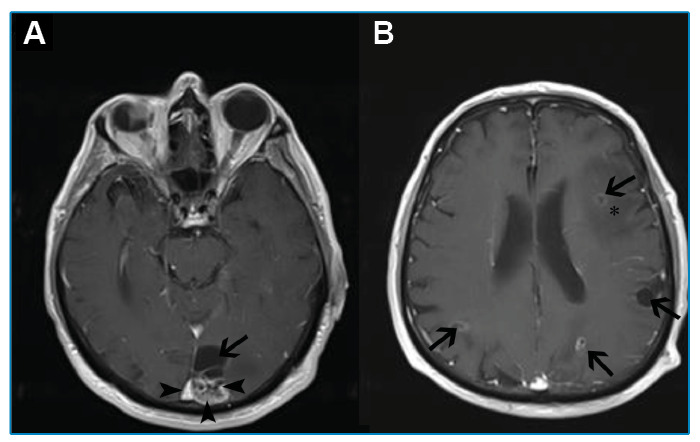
A. Axial post-contrast T1-weighted images show heterogeneous enhancement of the mural component (arrowheads) and irregular annular enchancement of the cyst wall in the occipital lobe lesion (arrow). B. Axial post-contrast T1-weighted images show multiple bilateral lesions (arrows) with surrounding edema (asterisks).
